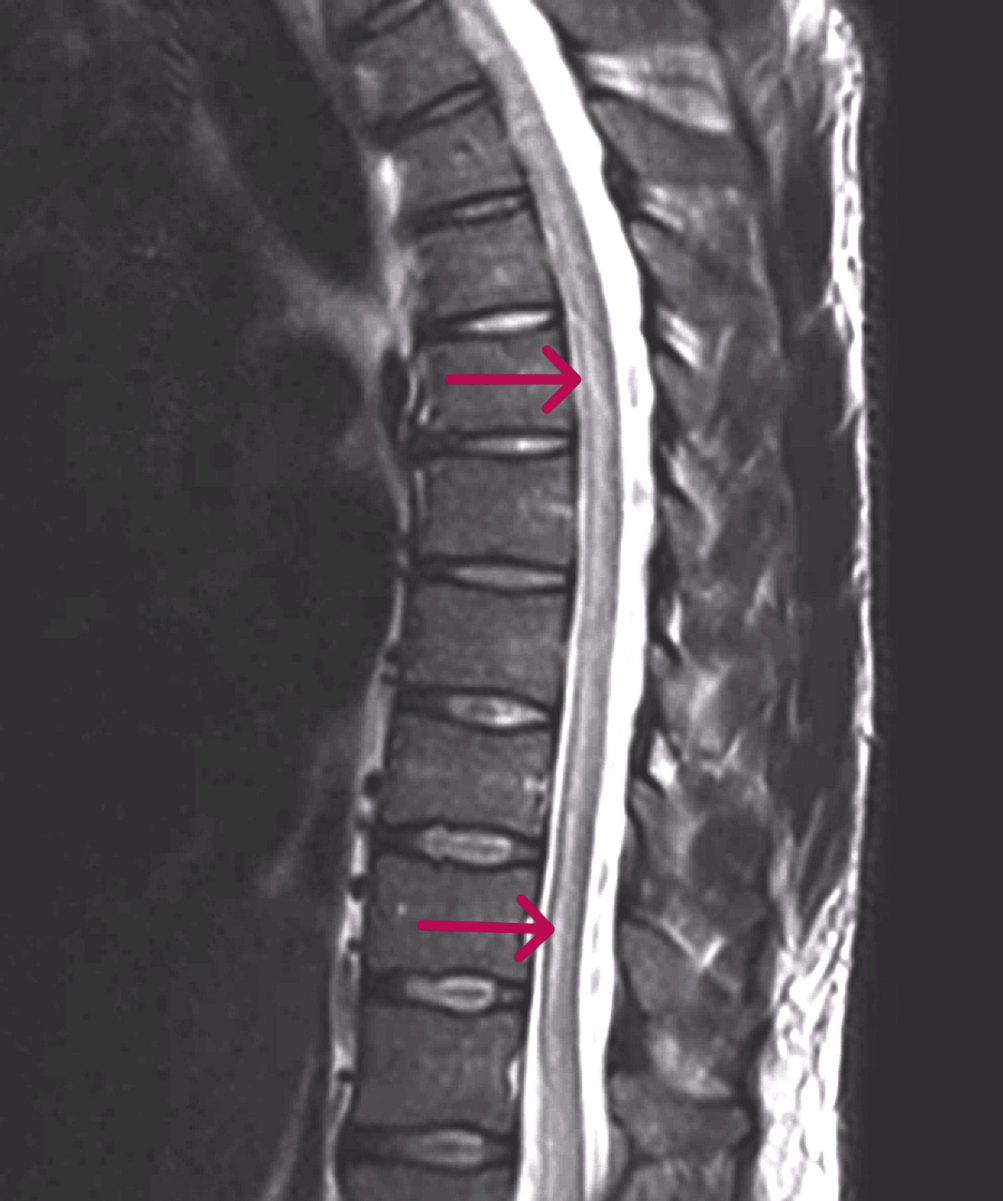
T2 weighted MRI of sagittal spine displaying gray matter lesion from T7 extending to conus medullaris. Arrows indicate involvement of the central gray matter
